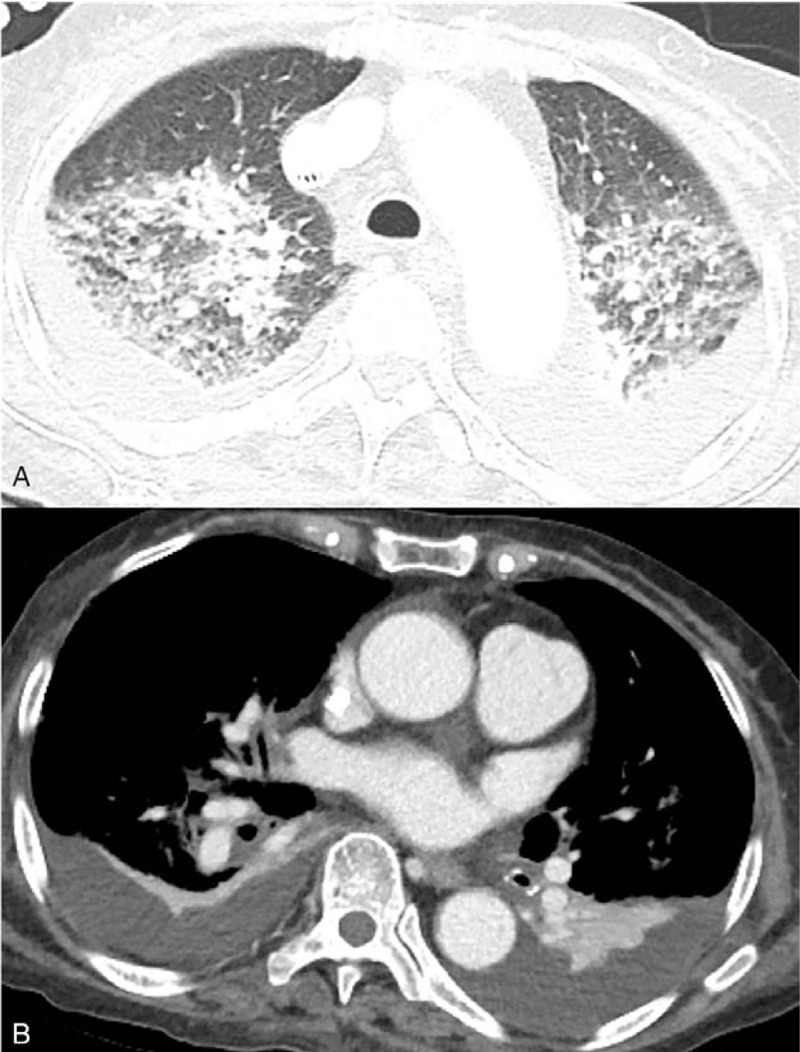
Chest computed tomography reveals patchy ground-glass opacities and consolidation with interlobular septal thickening in the dependent portion of the bilateral lungs (A). Moderate pleural effusion is noted in the bilateral lungs (B).
